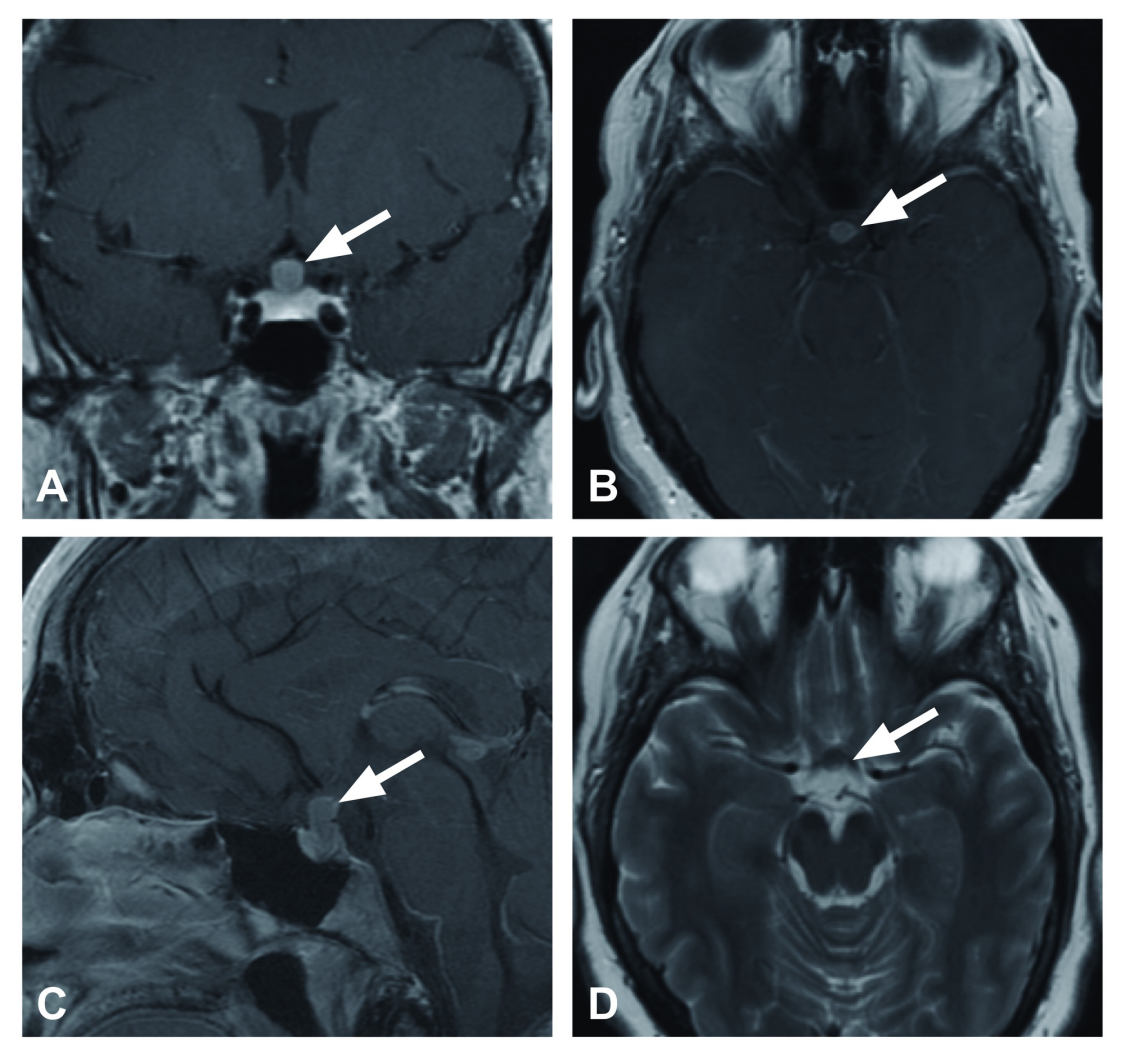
Preoperative magnetic resonance imaging. Preoperative magnetic resonance imaging demonstrates a hypoenhancing T1 and T2 intermediate lesion arising from the postero-superior aspect of the pituitary gland. The sellar-suprasellar lesion approximately 10 mm x 6 mm x 7 mm enhances with gadolinium administration. A) Coronal T1 post-gadolinium, B) axial T1 post-gadolinium, C) sagittal T1 post-gadolinium, D) axial T2.
